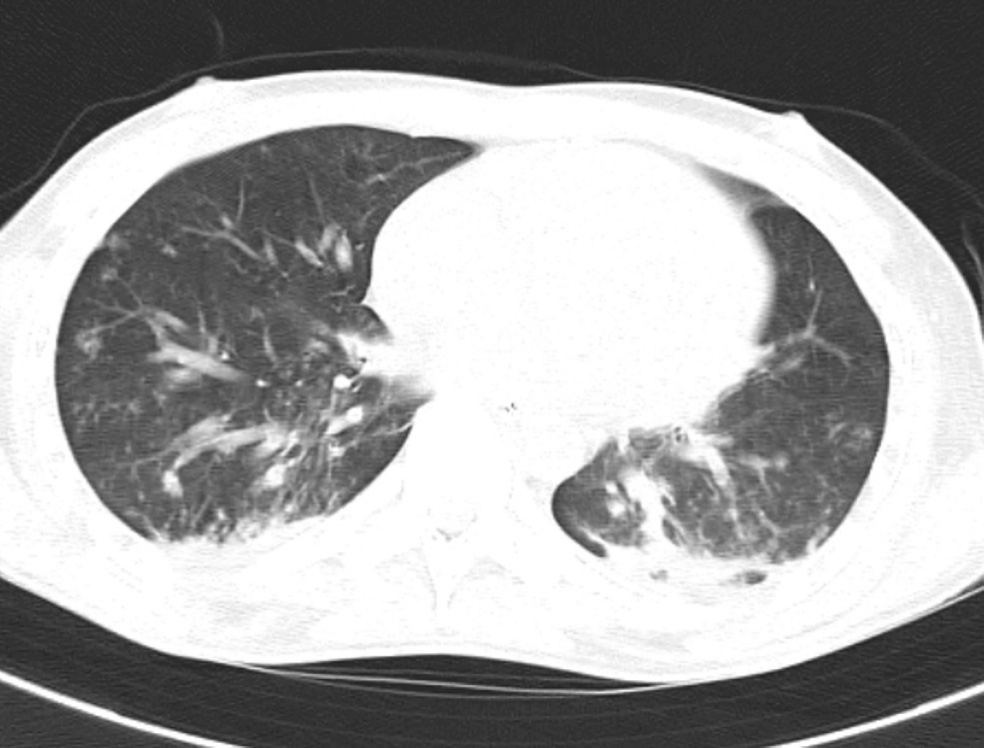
HRCT thorax shows bilateral posterior patchy consolidation. HRCT- High-resolution computed tomography
